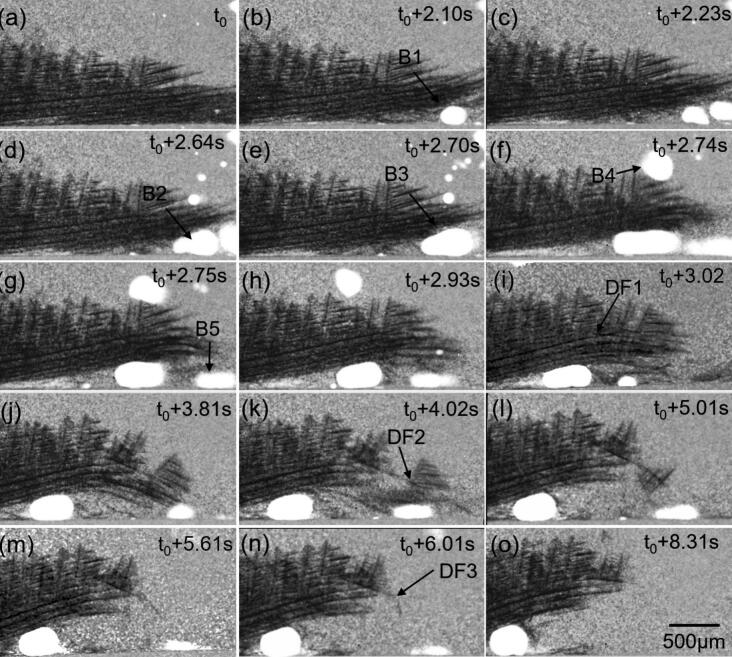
A series of X-ray images, showing multi-dendrites fragmentation induced by oscillating bubbles. Experimental conditions: 45 W ultrasound, 1137.5 K/m thermal gradient, 250 fps (see Video 6).
